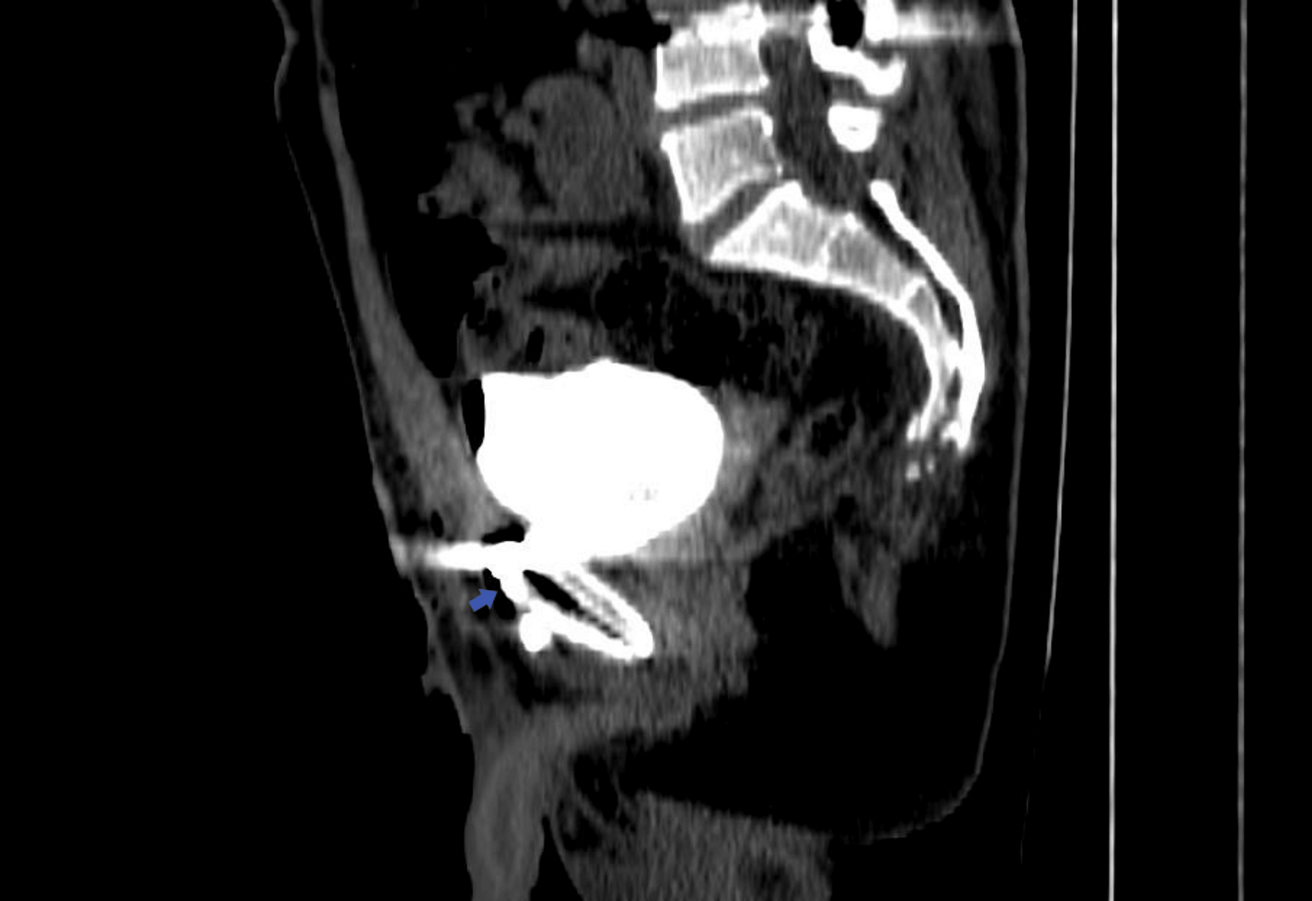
CT scan of the abdomen and pelvis, delayed phase, showing anterior bladder rupture with contrast extravasation (arrow) into retropubic space
